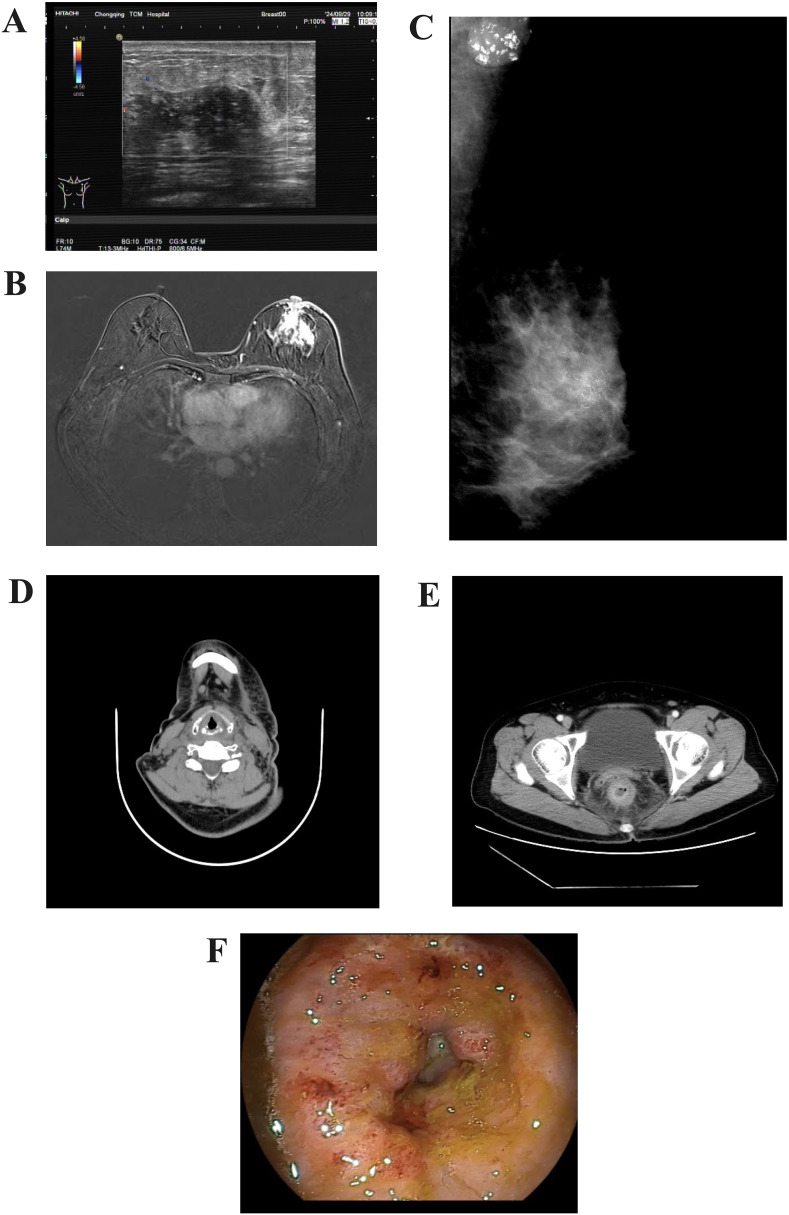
Imaging of the tumour. (A) Color Doppler ultrasound of the breast. (B) Contrast-enhanced MRI scan of the breast. (C) Mammography of the breast. (D) CT imaging of the cervical lymph nodes. (E) Contrast-enhanced CT scan of the lower abdomen. (F) Endoscopic appearance of the rectal lesion before treatment.
